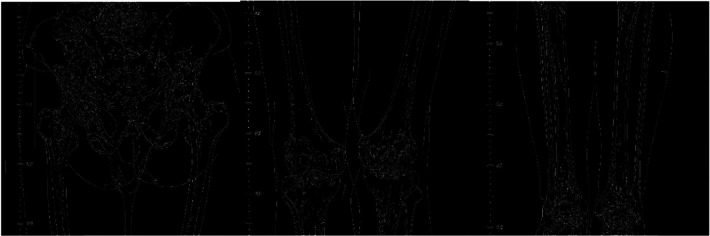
Three parts that consist the full-length lower limb x-ray image, the hip, the knee and the ankle, edges extracted by canny algorithm.
